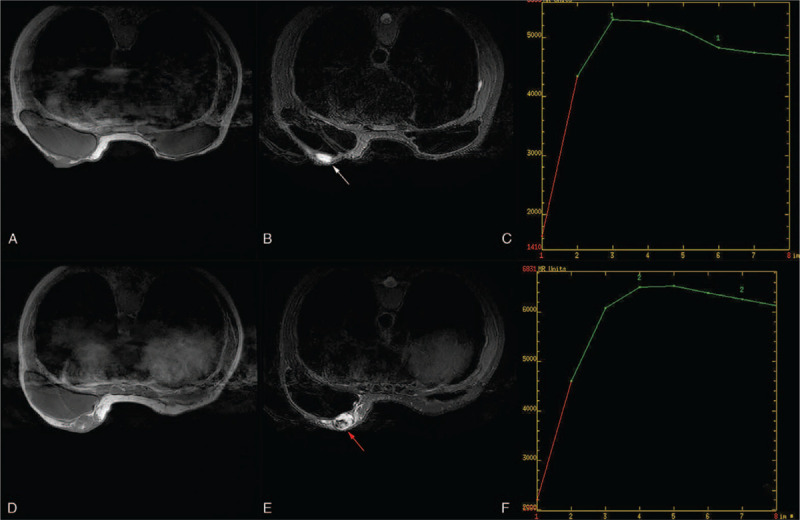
Magnetic resonance imaging of recurrent lesions of the left breast. Both the 2 lesions with (A & D) Low signal intensity on T1-weighted images. (B & E) High signal intensity on T2-weighted images. (C & F) Dynamic contrast-enhanced imaging with time signal intensity curves for type 3.
